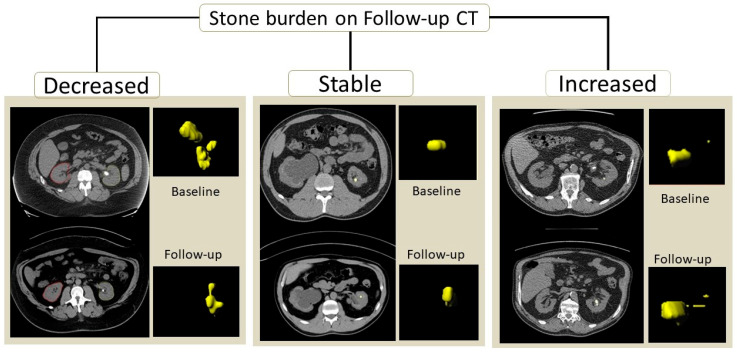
Transverse CT and “stone segmentation” volume-rendered images of three patients with decreased, stable, and increased stone burdens on a follow-up CT compared to the baseline CT.
